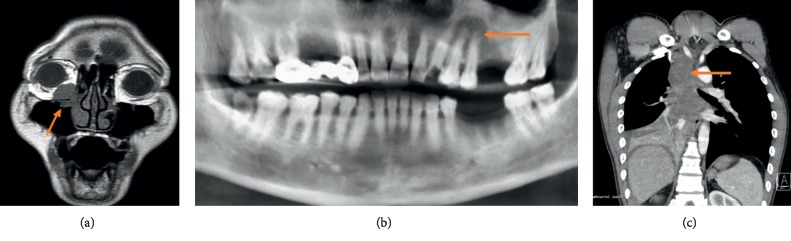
NUT carcinoma imaging characteristics. MRI scans revealing a 1 cm mass infiltrating the right orbit (a). CT scans revealing the presence of a 2 cm × 1.5 cm ovoid shadow at the apex of teeth 11-12 surrounded by dense white lines and an unclear boundary (b). CT scans indicating the presence of an area of soft tissue of irregular density within the right lung hilum, with contrast-enhanced images revealing the presence of nonhomogeneous enhancement and local necrosis (c).
